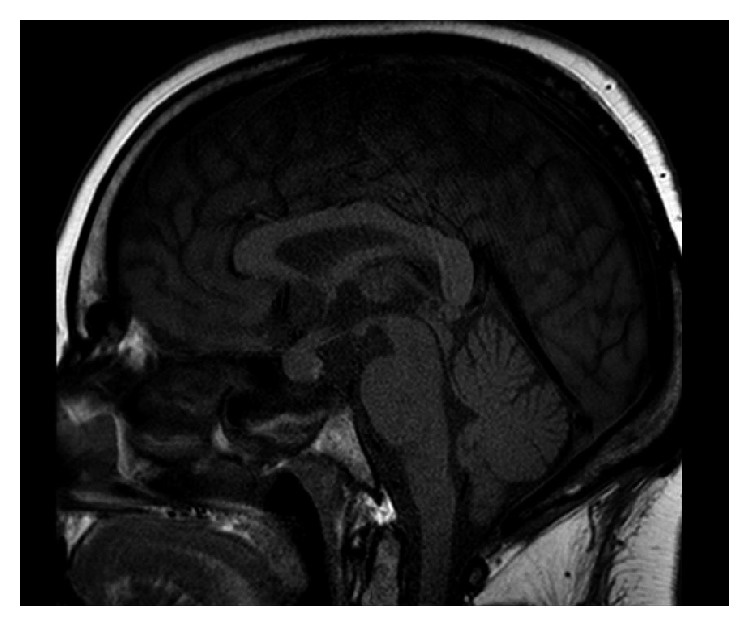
Brain MRI showing enlargement of the pituitary gland and pituitary stalk without evidence of adenoma.
